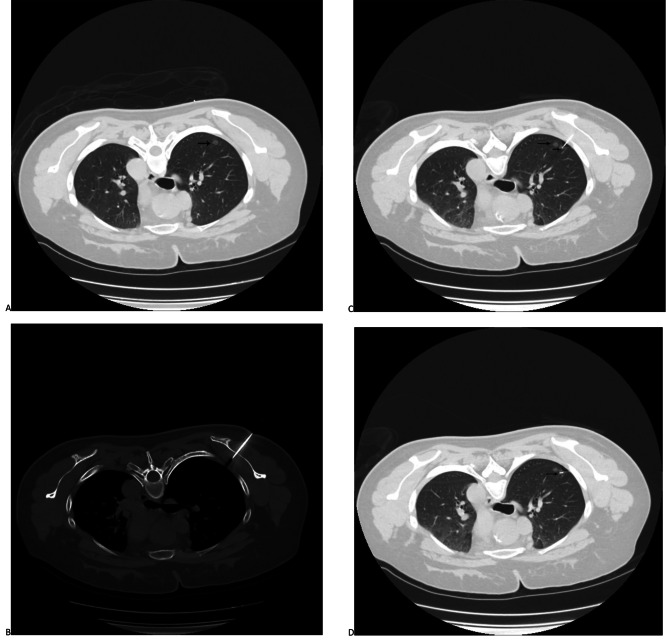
The procedures of the trans-scapula SCN localization. (a) Preoperative CT for the PN (arrow) behind the scapula; (b) The scapula was punctured; (c) The tip (short arrow) of the SCN was advanced near the PN (long arrow). (d) The anchor claw (arrow) of the SCN was released near the PN
